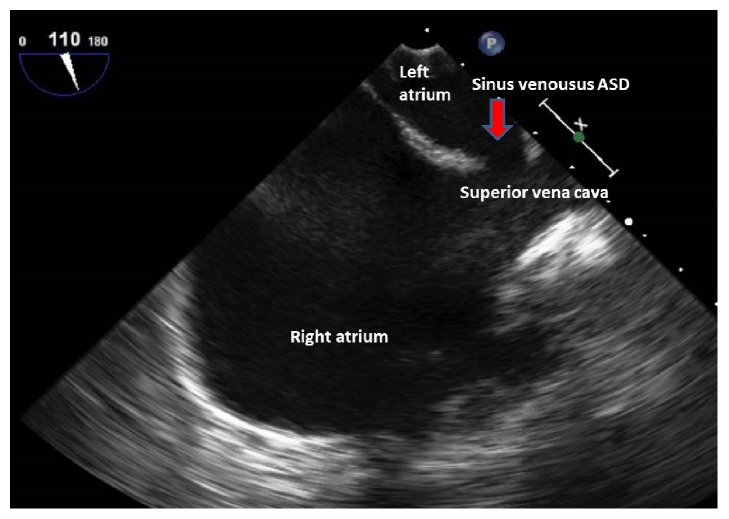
A bicaval view via a transesophageal echocardiogram shows communication (red arrow) between the left atrium and the superior vena cava entry.
